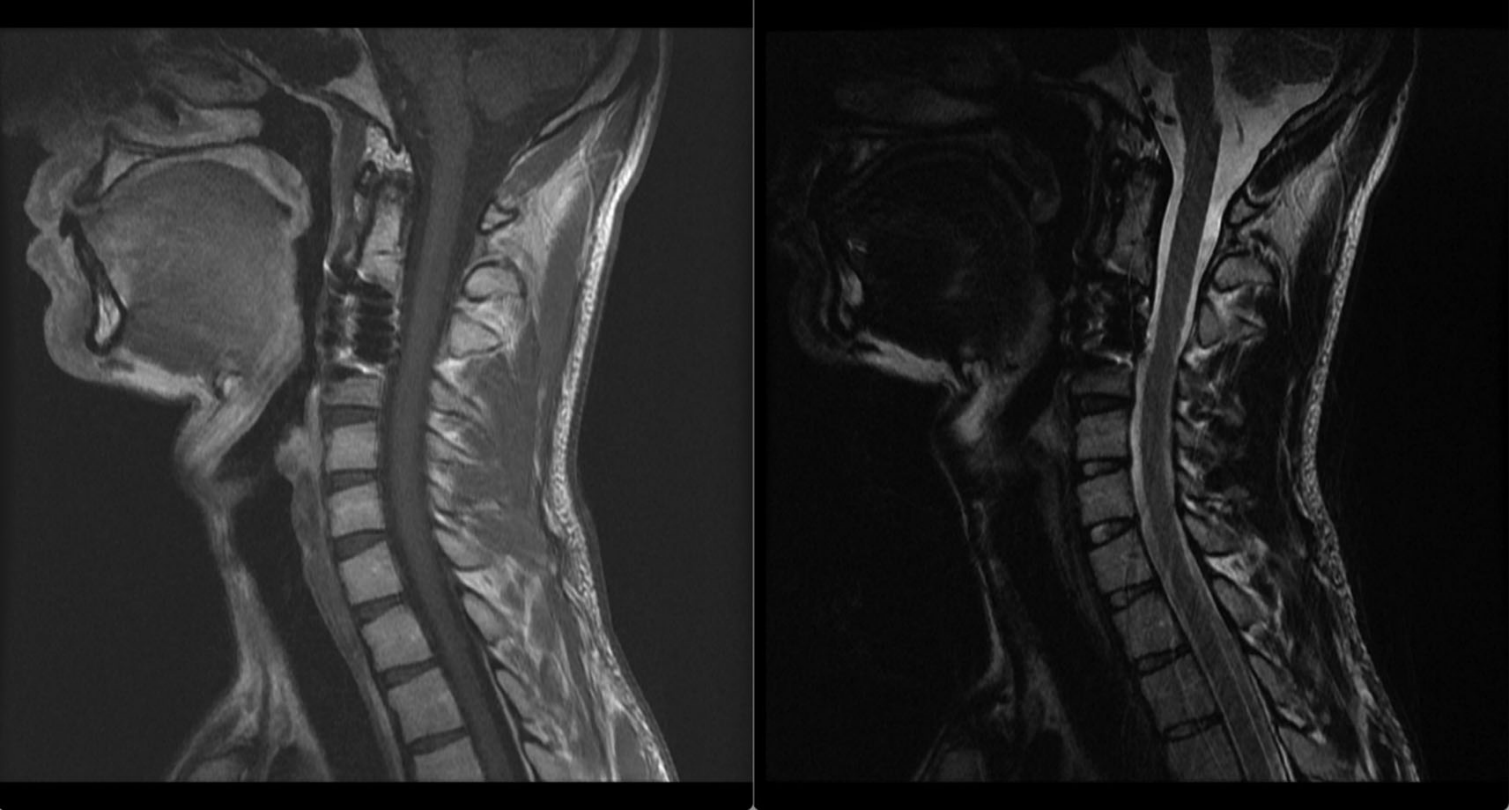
One month after surgery, follow-up MRI scans showed no evidence of tumor growth in the C3 vertebral body.
